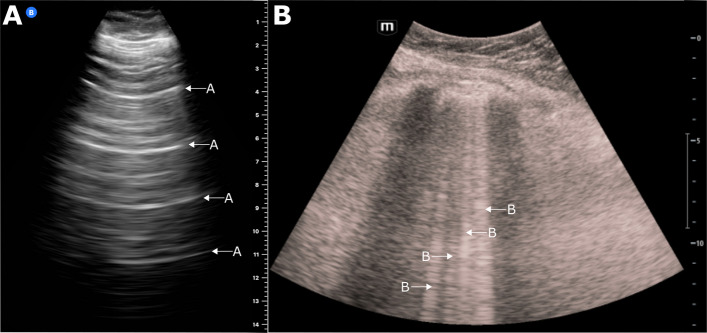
Common lung point-of-care ultrasound (POCUS) findings. A Normal lung POCUS without evidence of pulmonary edema. Arrows with the letter A identify A-lines, or normal artifacts representing reverberation of the pleura. B Lung POCUS indicating greater than 3 B-lines (identified by arrows with the letter B), which is diagnostic of pulmonary edema
